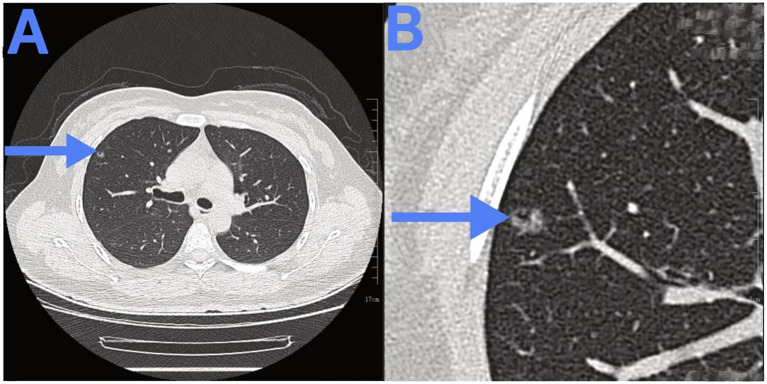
Representative comparison of image quality and nodule visualization between conventional HRCT and ultra-high-resolution CT (UHRCT) in the same patient. (A) Conventional HRCT image (512 × 512 matrix) showing a ground-glass nodule (GGN) in the right lung (blue arrow). Note that the internal structure and marginal characteristics appear relatively blurred due to the standard resolution. (B) UHRCT image (1024 × 1024 matrix) of the same lesion with a targeted field of view. The increased spatial resolution significantly enhances the visualization of morphological details. The blue arrow points to the nodule, revealing sharper margins and clearer internal features compared to (A). Pathological diagnosis: The postoperative pathology confirmed this nodule as Minimally Invasive Adenocarcinoma (MIA). HRCT, high-resolution computed tomography; UHRCT, ultra-high-resolution computed tomography.
